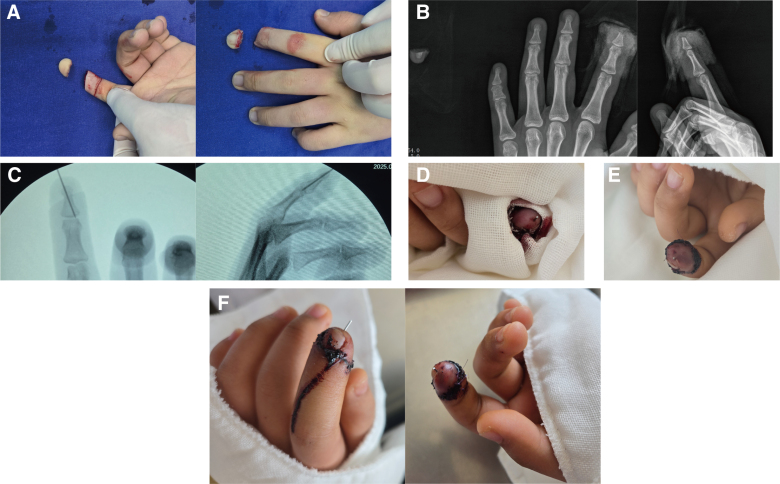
Preoperative photograph (A) and radiographs (B) of the left index finger showing an oblique amputation at the distal phalanx level (Tamai zone I). (C) Intraoperative radiograph demonstrating Kirschner-wire fixation. (D) Postoperative photograph taken at 2 hours demonstrating restoration of circulation in the replanted fingertip, with improved skin color and capillary refill. (E) Postoperative photograph taken at 3 days showing a purplish discoloration of the replanted fingertip with delayed capillary refill. (F) Postoperative photograph taken on postoperative day 7 (day of discharge) showing good circulation in the fingertip with normal color and brisk capillary refill.
